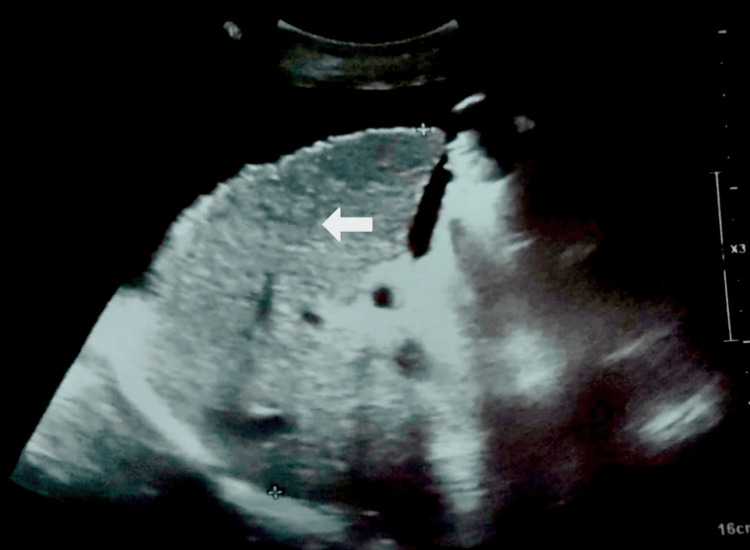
Right upper quadrant grayscale ultrasound image showing multiple hypoechoic nodules within the liver parenchyma on a background of coarse echotexture, findings suggestive of cirrhosis with nodular regenerative changes. The white arrow indicates the hypoechoic nodules
